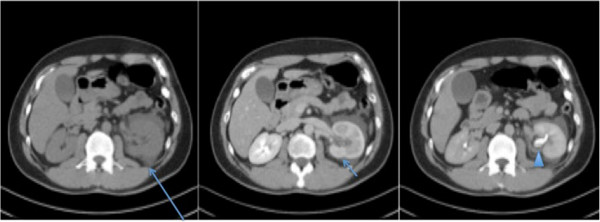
Axial computed tomography image in the plain, nephrographic and pyelographic phases. A large amount of perinephric fluid is seen on the left side (long arrow in first panel). Delayed enhancement of the left kidney with delayed excretion of contrast is apparent (short arrow in second panel), but no solid mass or urinary calculus is seen. Dependent material is seen in the nondilated collecting system of the left kidney consistent with blood (arrowhead in third panel).
